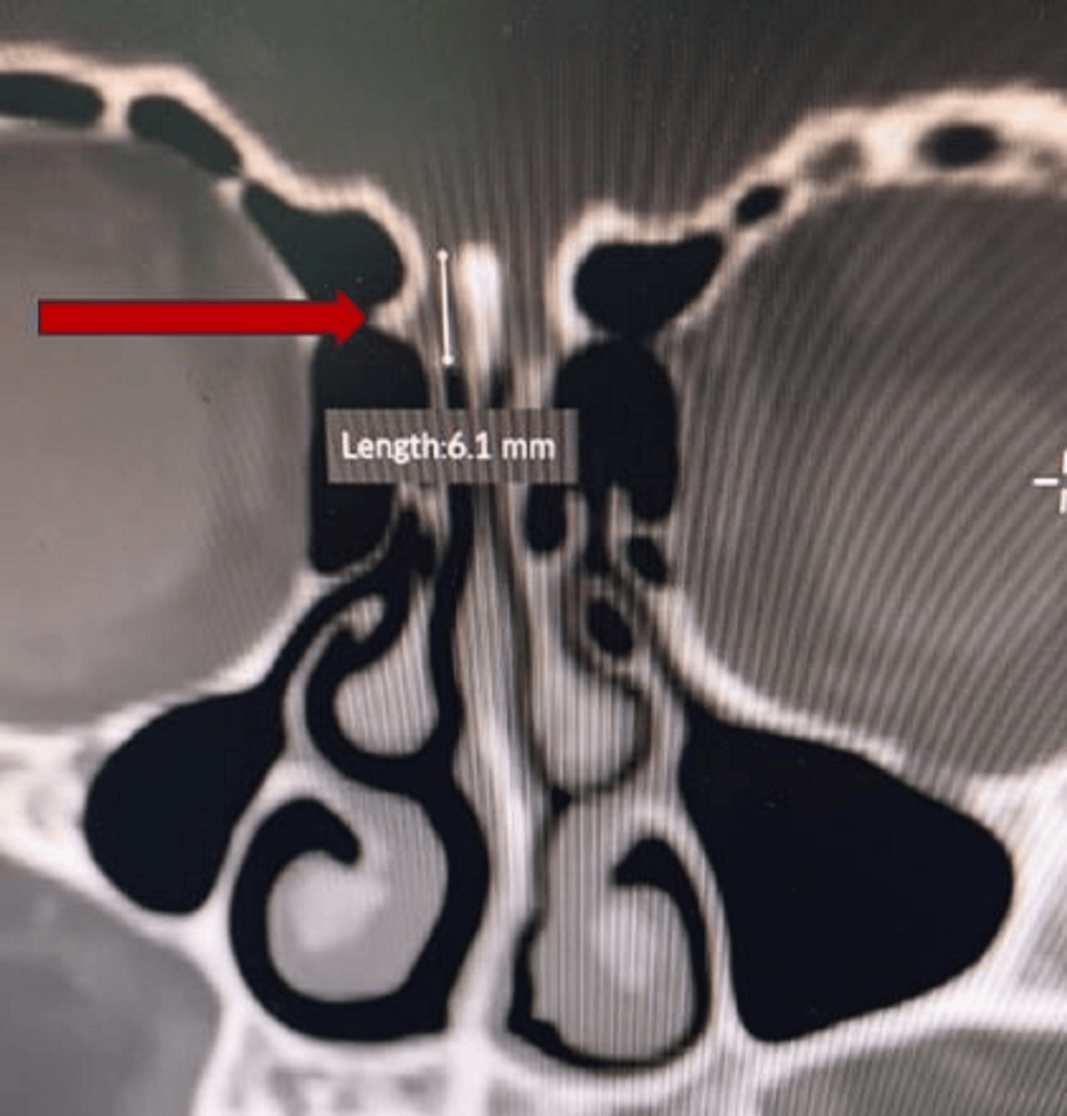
Coronal section of CT scan paranasal sinuses showing right sided type 2 Keros
